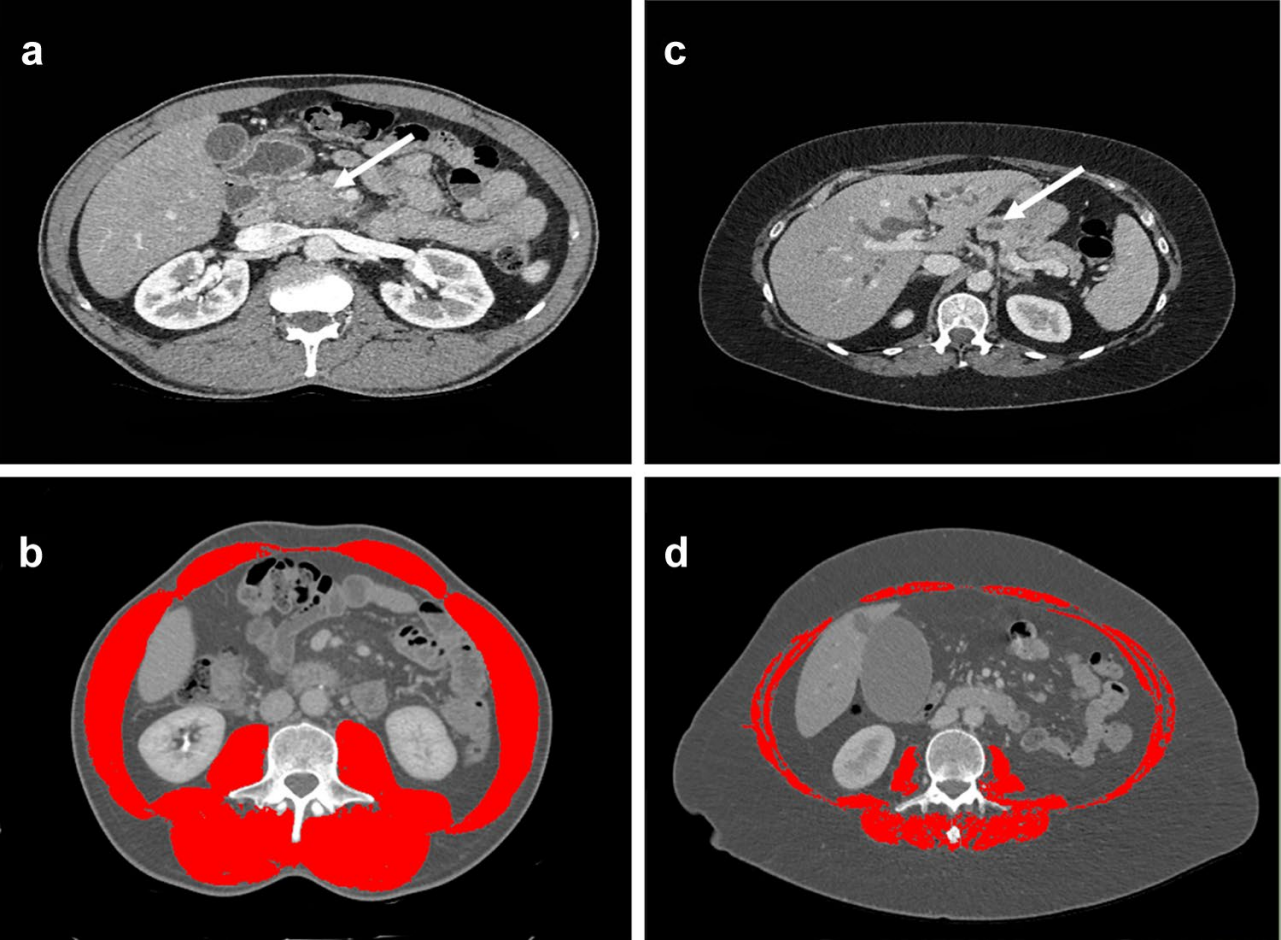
Computed tomographic skeletal muscle analysis in patients with locally advanced pancreatic cancer. Patient 1: a locally advanced tumour visible in the uncinate process (white arrow), despite patient's relatively low BMI (21.6 kg/m^2^) computed tomographic muscle mass analysis at the L3 level, (b) showed a normal ratio of skeletal muscle (highlighted red) SMI 50.1 cm^2^/m^2^ with the patient receiving chemotherapy. Patient 2: c with a locally advanced pancreatic cancer in the head and a dilated main pancreatic duct (white arrow), despite the high BMI 32.1 kg/m^2^ computed tomographic muscle mass analysis demonstrated a low SMI 31.3 cm^2^/m^2^ consistent with sarcopenia, the patient did not go on to receive chemotherapy. The presence of a high BMI > 25 kg/m^2^ and sarcopenia is termed ‘sarcopenic obesity’ [24]
